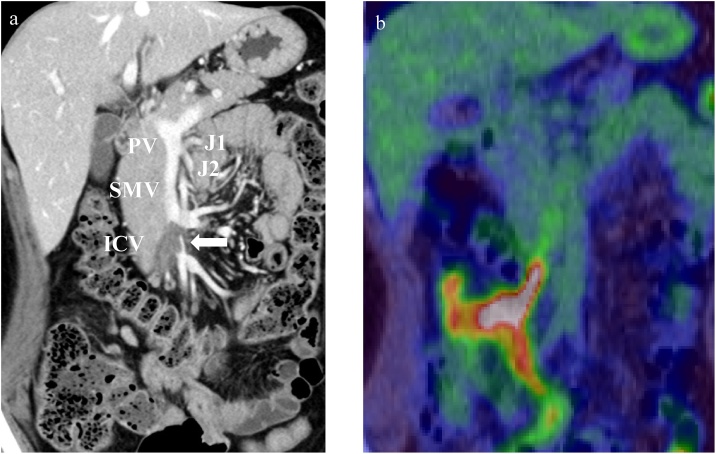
Images of the tumor thrombosis. (a) Contrast-enhanced CT shows an intraluminal filling defect from the ileocolic vein to the superior mesenteric vein (SMV; white arrow). (b) On PET-CT, abnormal FDG uptake extended from the ileocolic vein (ICV) to the SMV (SUV-max: 9.4).
